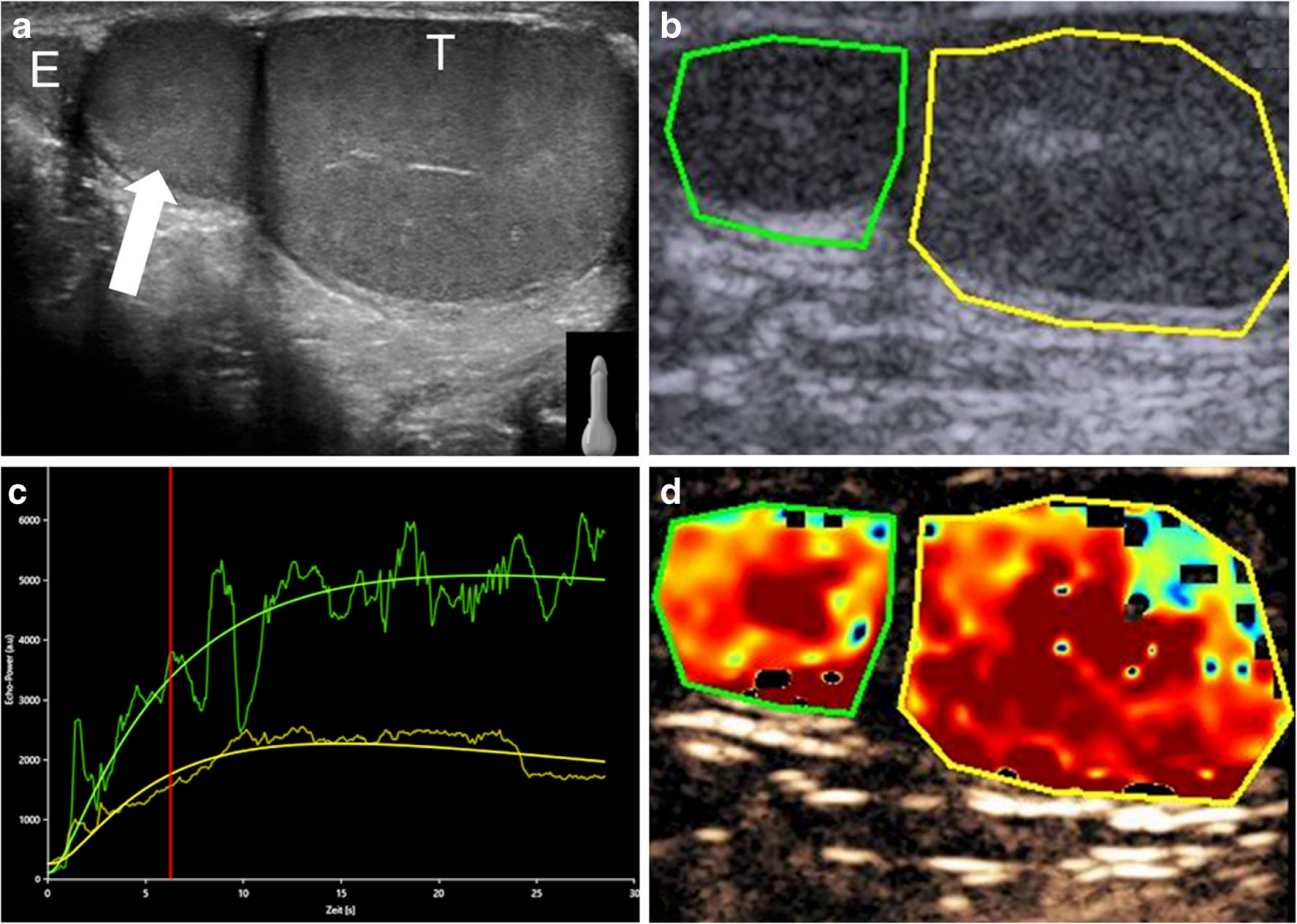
Palpable lesion adjacent to the right testicle in an 11-year-old boy. a Longitudinal gray-scale US of the right testis shows a round extra-testicular structure (*arrow*) between the epididymis (*E*) and the right testis (*T*), with similar reflectivity as the adjacent testicular tissue. b, c Quantitative contrast-enhanced ultrasound (CEUS) with time-intensity curve analysis, with a region of interest placed within the right testis (*yellow circle* in b) and the structure of concern (*green circle* in b). Time-intensity curve analysis of contrast dynamics (c) shows a similar wash-in and curve progression in the first 30 s for both structures. d Transverse CEUS color-coded map shows similar enhancing parameters for the structure of concern and the right testis. This combination of imaging findings favors the diagnosis of supernumerary testis compatible with polyorchidism
